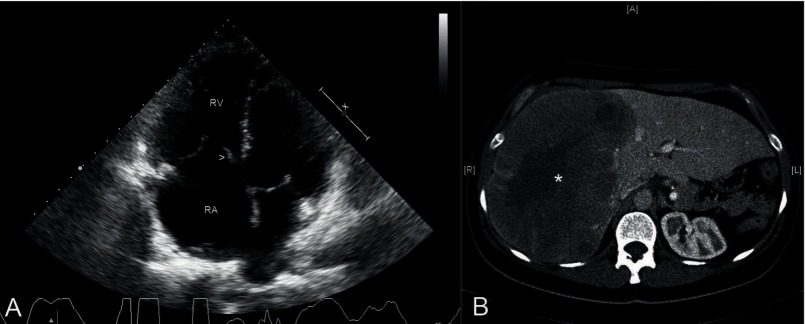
Echocardiography and Abdominal Computed Tomography A: Two-dimensional apical 4-chamber echocardiographic view showing a thickened and fixed tricuspid septal valve (arrow head) that does not allow proper coaptation of the tricuspid valve. RA: right atria, RV: right ventricle. B: Abdominal computed tomography with contrast showing hepatomegaly and a large mass in the right hepatic lobe (asterisk).
